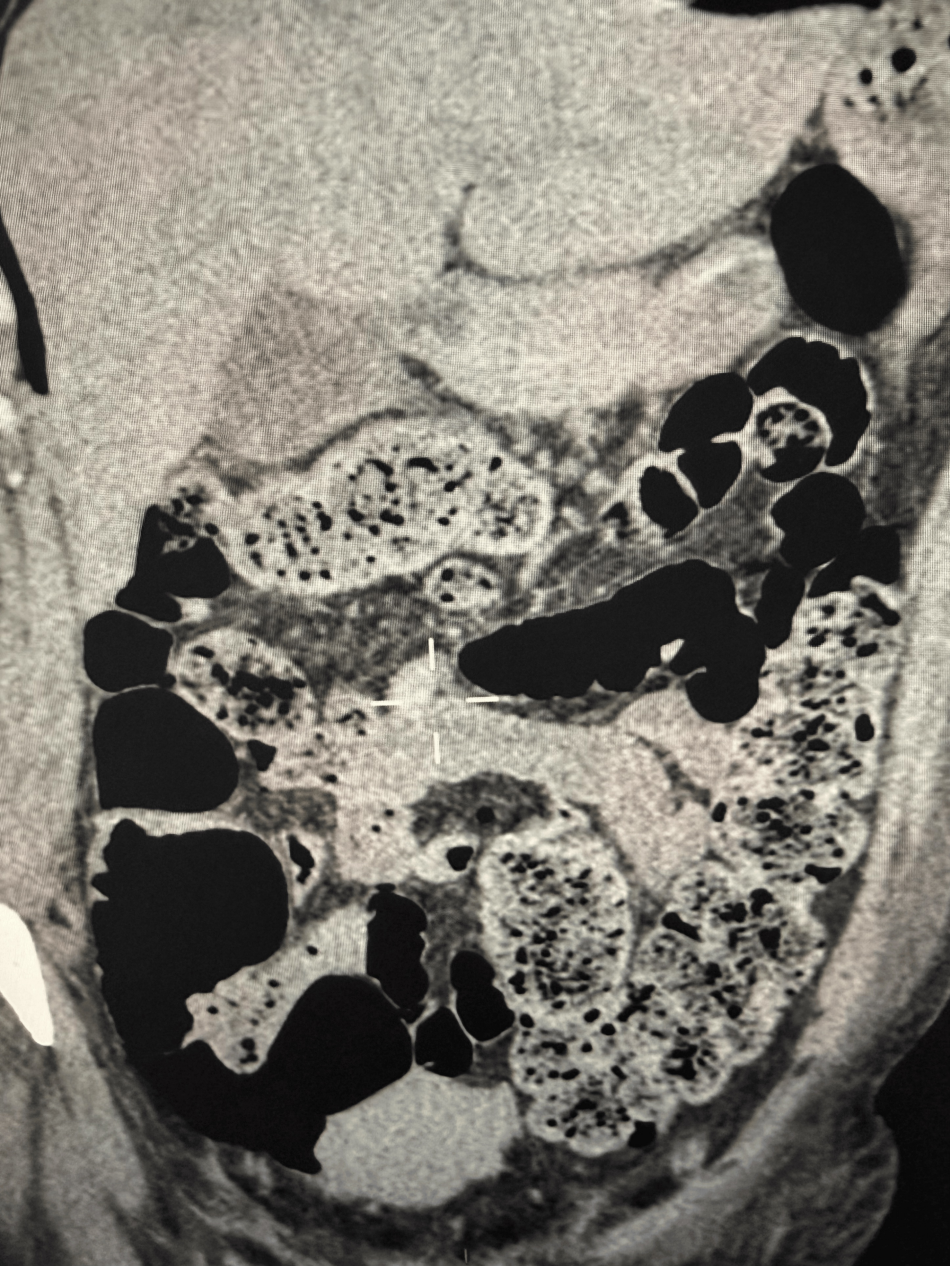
Urgent contrast-enhanced computed tomography (CT) of the abdomen revealing free intraperitoneal air, pericolonic inflammation, and bowel wall thickening
